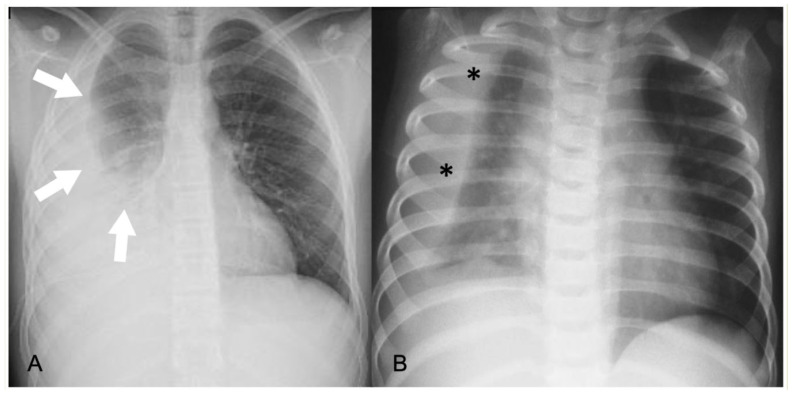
Pleural effusions on chest radiographs: (A) meniscus sign (arrows); (B) loculated effusion with convex borders (*) towards the spine.
